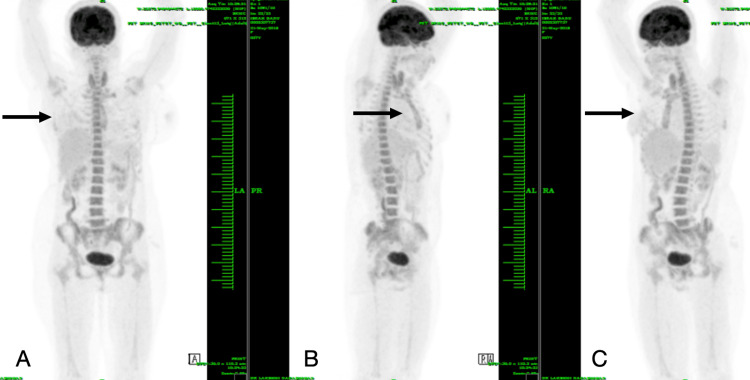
Postoperative PET-CT image showing no uptake in the region of the tumor. (A) Coronal view; (B) sagittal view; (C) oblique view. Arrows indicate the preoperative location of the tumor in the different views PET-CT: positron emission tomography-computed tomography
